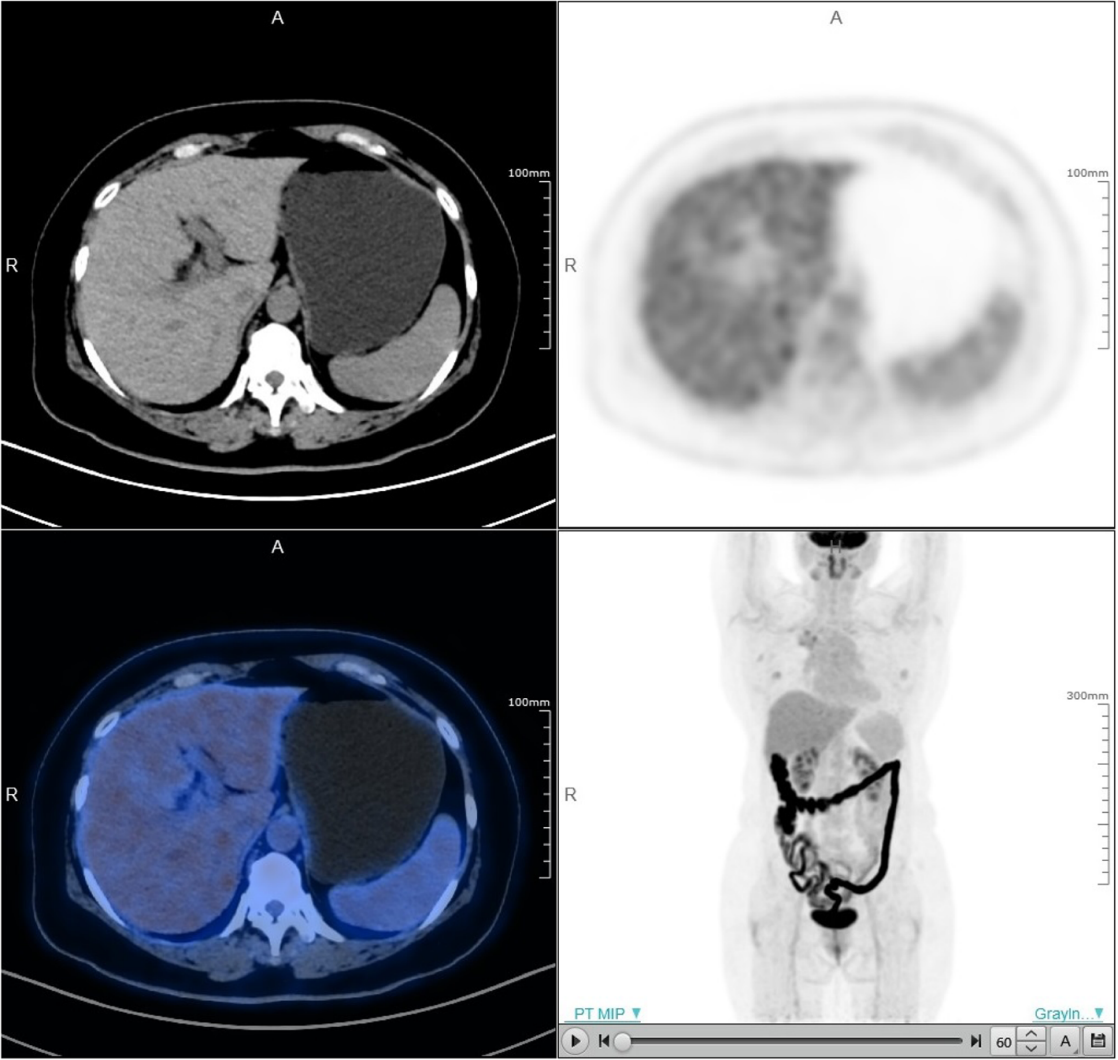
Third patient images, upper left is CT image, upper right is PET image, lower left is PET/CT fusion image, and lower right is MIP image
